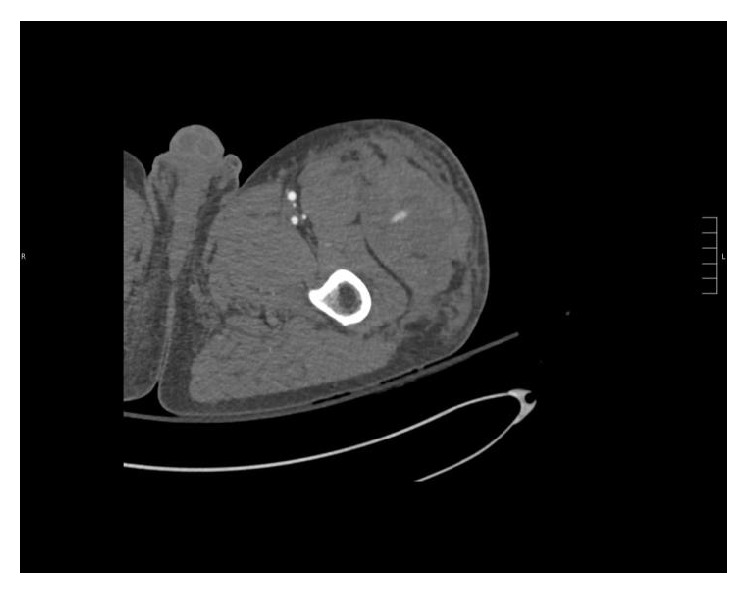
Contrasted enhanced CT of the left thigh demonstrates an area of extravasation and surrounding hematoma.
